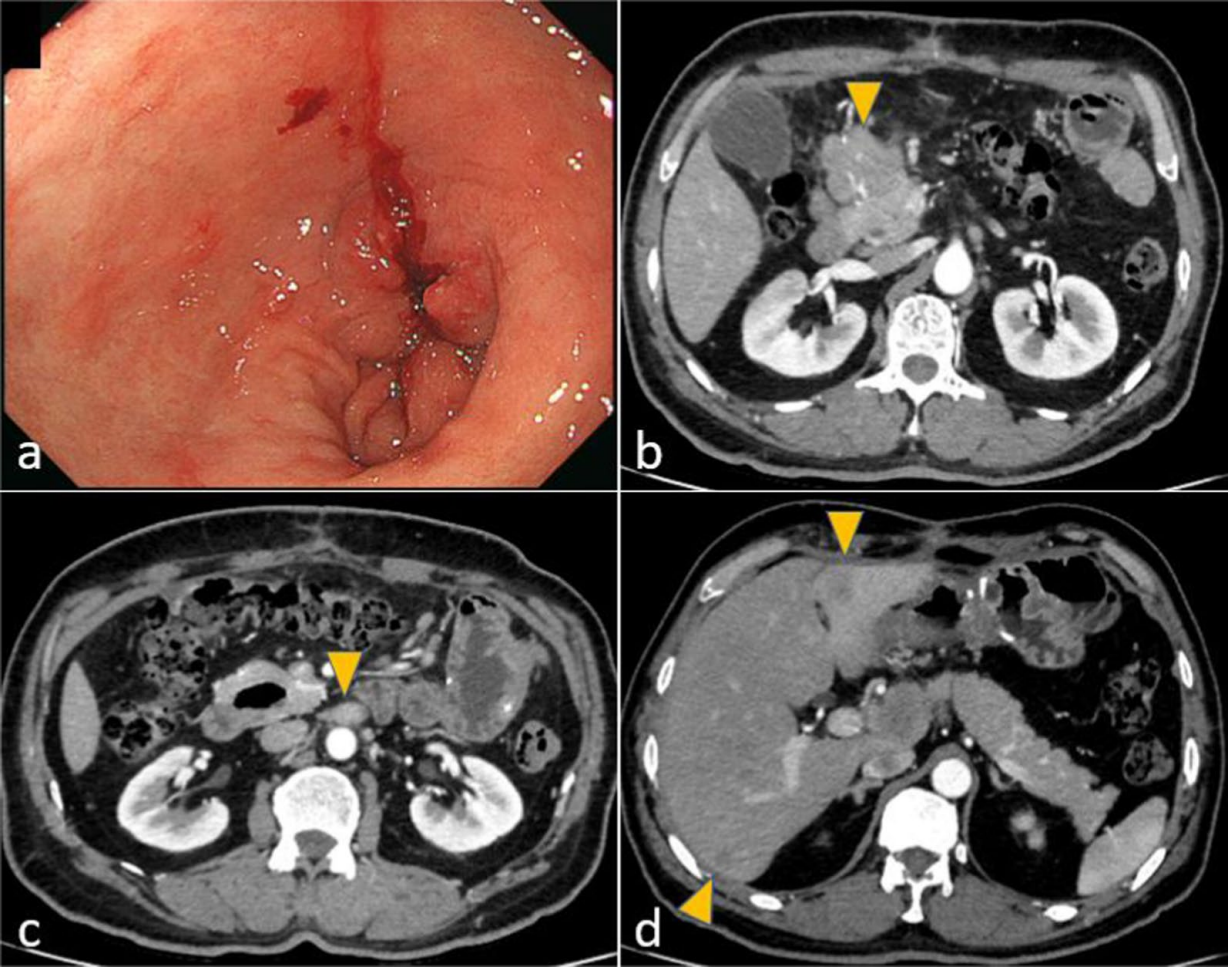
Findings of case 3 with gastrointestinal endoscopy and abdominal computed tomography. a A type 3 tumor caused circular stenosis from the antrum to the pylorus. b, c Station No. 6 and 16b1 lymph nodes were enlarged. The station No. 6 lymph node had invaded the pancreatic head. d Two small liver metastases were newly found during chemotherapy
